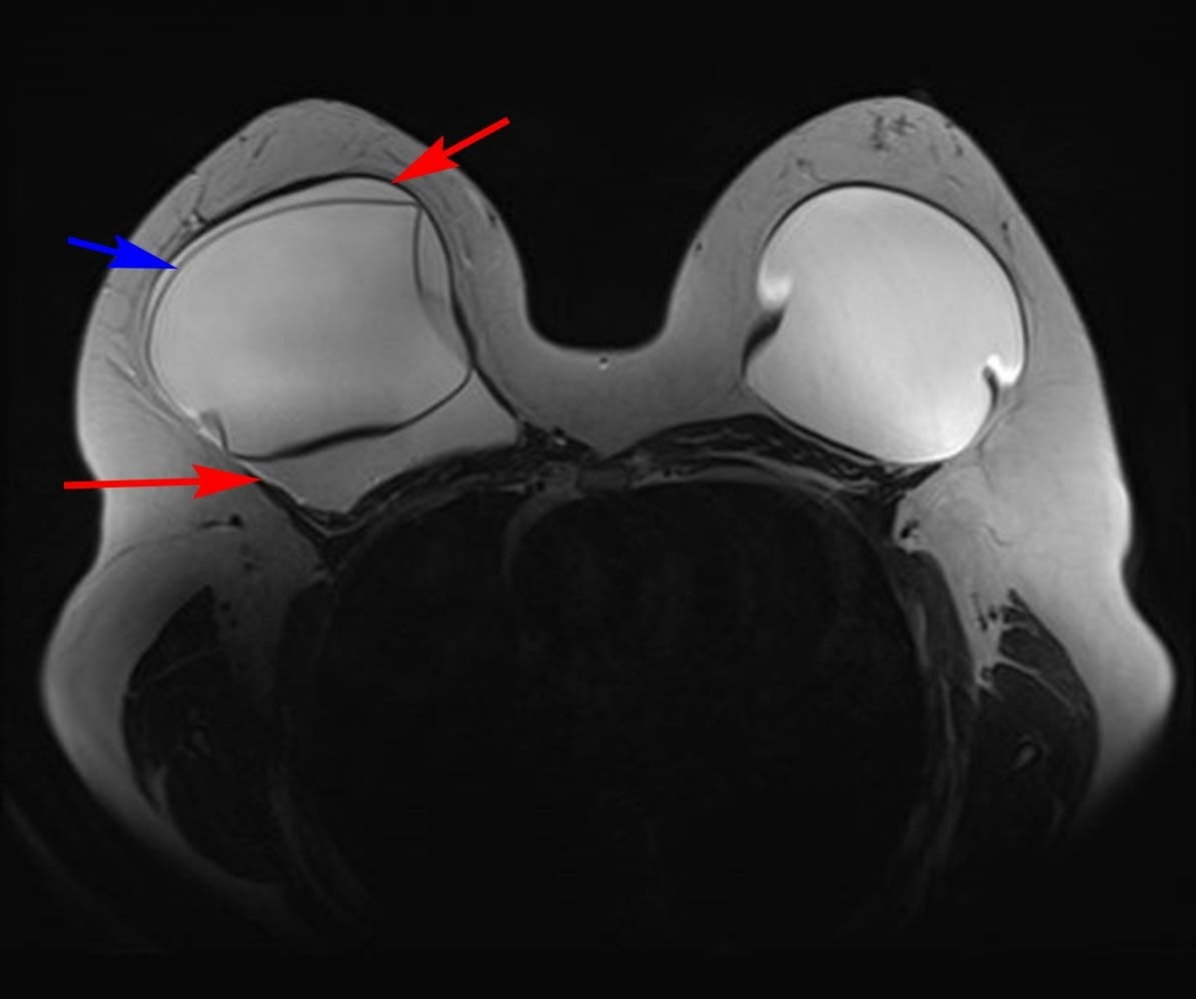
Axial MRI T2 weighted image of the bilateral breasts A T2 hyperintense collection (red arrows) surrounds the implant in the right breast. The implant capsule (blue arrow) is intact.
